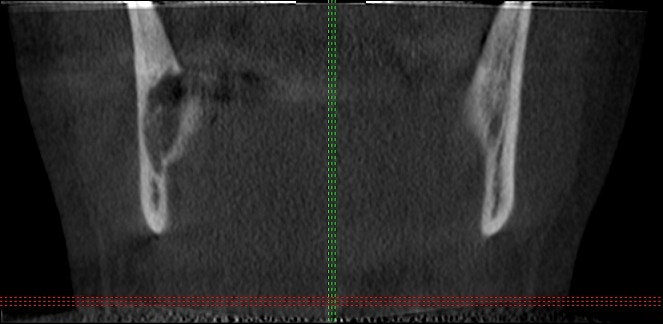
Cone beam computed tomography (CBCT) image of the lesion
